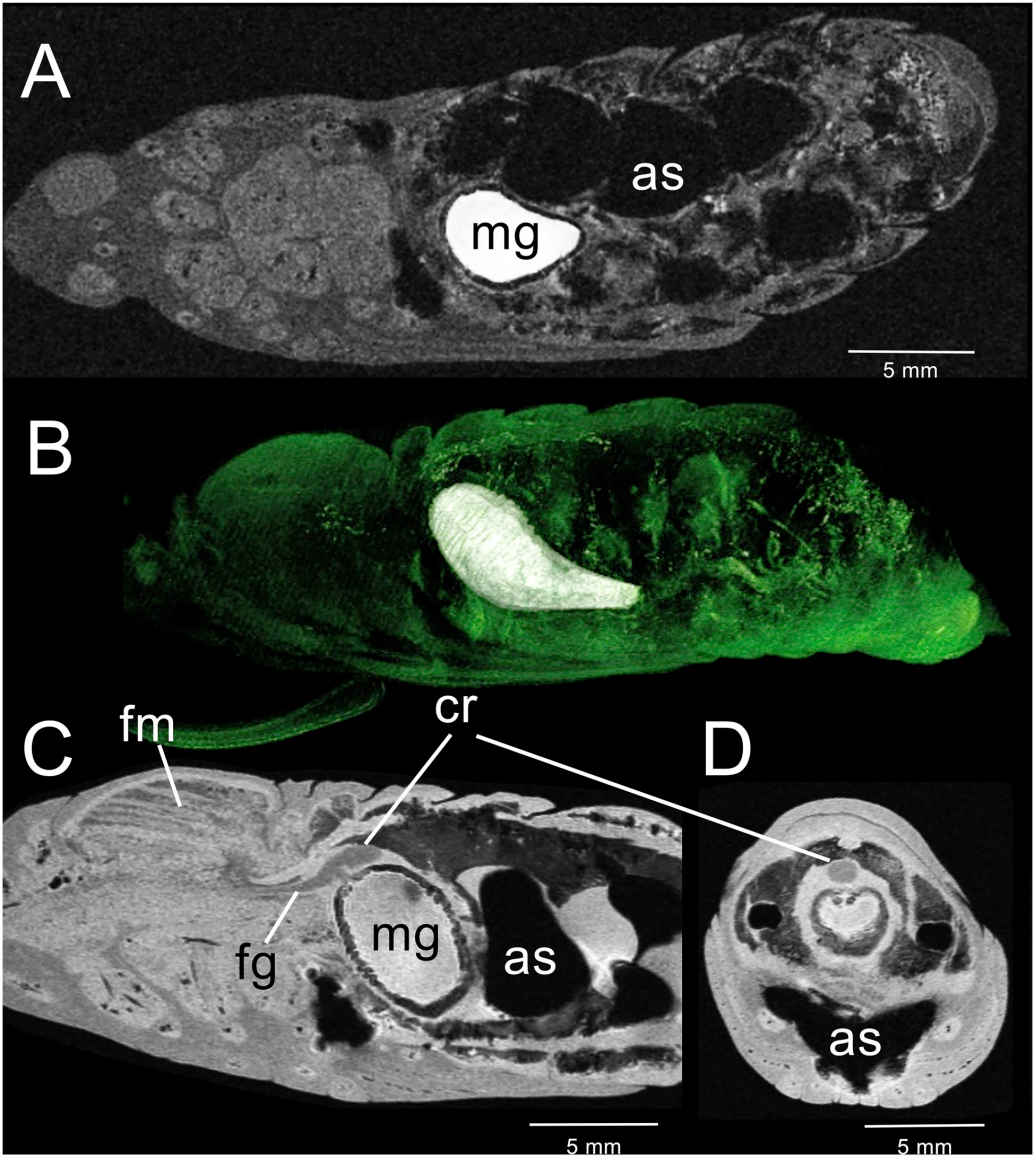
MR imaging data acquired from a *M*. *sexta* pupa 5–7 days after metamorphosis. (A) 3D-GRE coronal section displaying the enlarged air sacs (as). (B) Sagittal volume rendering view displaying the position of the midgut (mg, containing contrast agent) and developing hindgut (not containing contrast agent). (C) 3D-FSE sagittal section displaying the developing thoracic flight muscles (fm), midgut and crop (cr). The newly formed crop expands from the foregut (fg). (D) Transverse section through the crop and anterior midgut.
